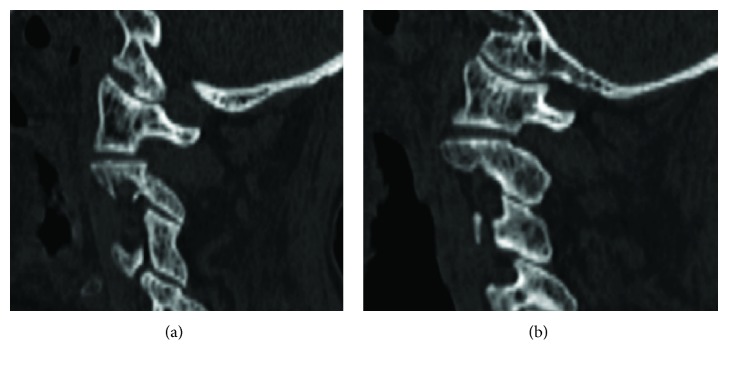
Left (a) and right (b) parasagittal CT scan images demonstrate complete reduction of both the atlantooccipital joint and the atlantoaxial joint.
